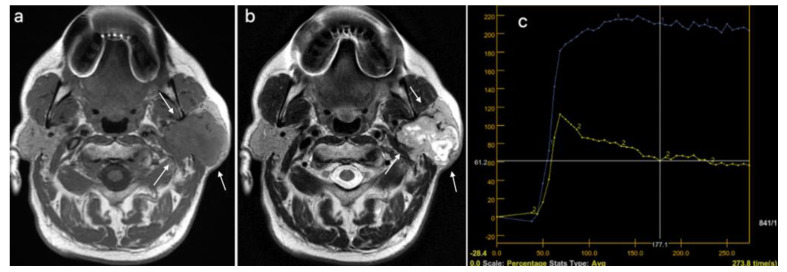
Pleomorphic adenoma of the left parotid gland (white arrows): (a) T1WI sequence showing a hypointense signal in the lobulated lesion, well delineated; (b) T2WI sequence showing an inhomogeneous, predominantly hyperintense signal in the tumor, with central cystic areas; (c) DCE-MRI showing a type A TIC curve.
